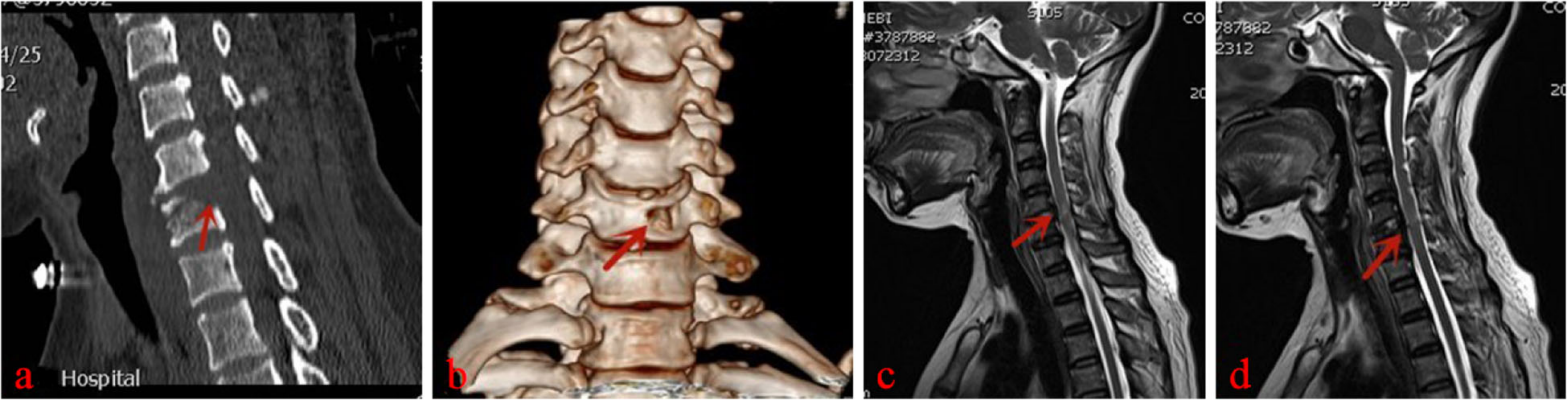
Postoperative CT showing that the disc-osteophyte complex had been removed, three-dimensional reconstruction showing the complete vertebral body tunnel, the vertebral body height is not lost, and no fracture occurs (a, b). The spinal cord decompression was sufficient on MRI examination(c, d)
